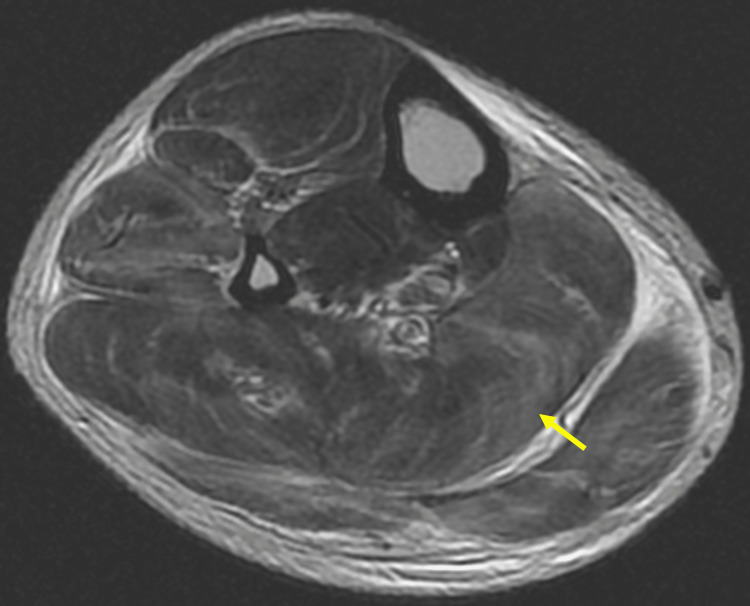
Axial section T2-weighted sequence of the right lower limb. Axial section T2-weighted sequence of right lower limb showing extensive intramuscular hyperintensities in the anterior compartment (extensor digitorum longus), lateral (peroneus longus), and superficial posterior compartment (plantaris, gastrocnemius, and soleus) and intermuscular facial planes, predominantly in the proximal part. There are no e/o necrotic changes.
